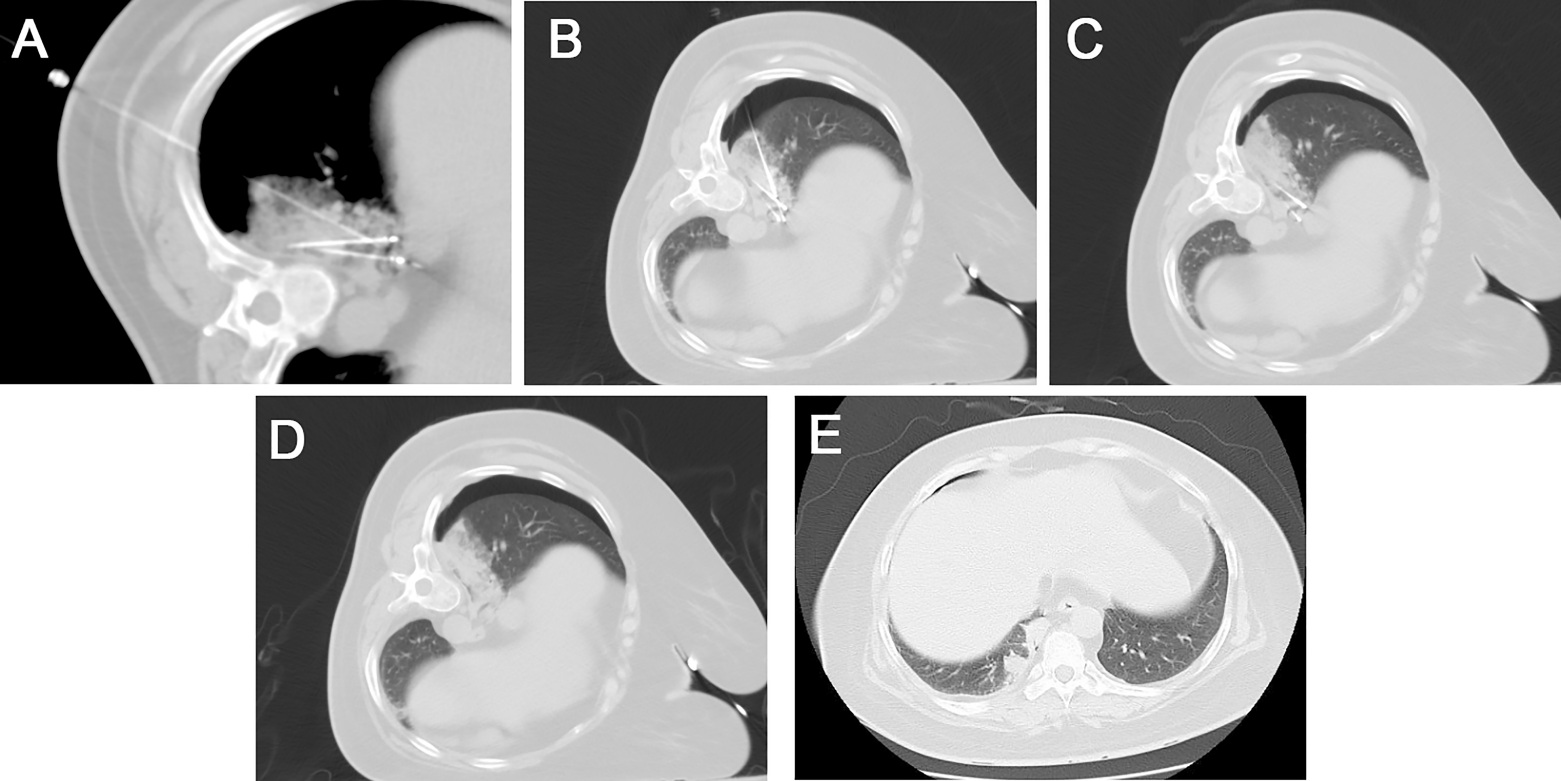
(A) Under CT guidance, the radiofrequency ablation probes (long arrow) were placed for the two lesions, and local nerve block anesthesia (short arrow) was performed using a 21G Chiba needle to the diaphragm. (B) A semi-automatic biopsy was used to obtain the tumor tissue from one of the lesions. (C) A scan was performed after 12 min of ablation with a power of 30 w, showing the formation of a halo sign around the lesion. (D) After removing the needle and probes, the CT scan showed substantial localized changes in the ablation zone. A small amount of bleeding from the puncture needle tract with a small amount of pneumothorax on the right side. (E) A follow-up one week after the operation showed a well-defined solid area in the ablation zone.
